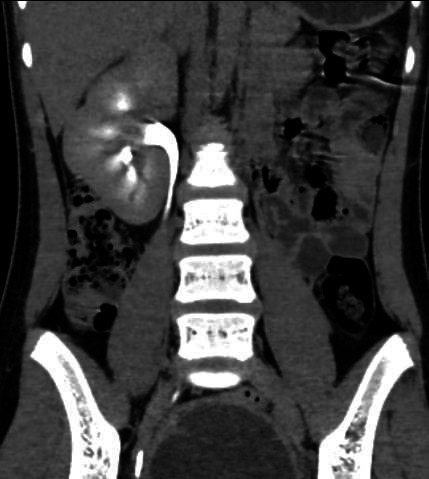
A delayed phase of contrast-enhanced CT scan of abdomen and pelvis showing associated left renal agenesis. The right kidney and pelvicalyceal system are normal.
